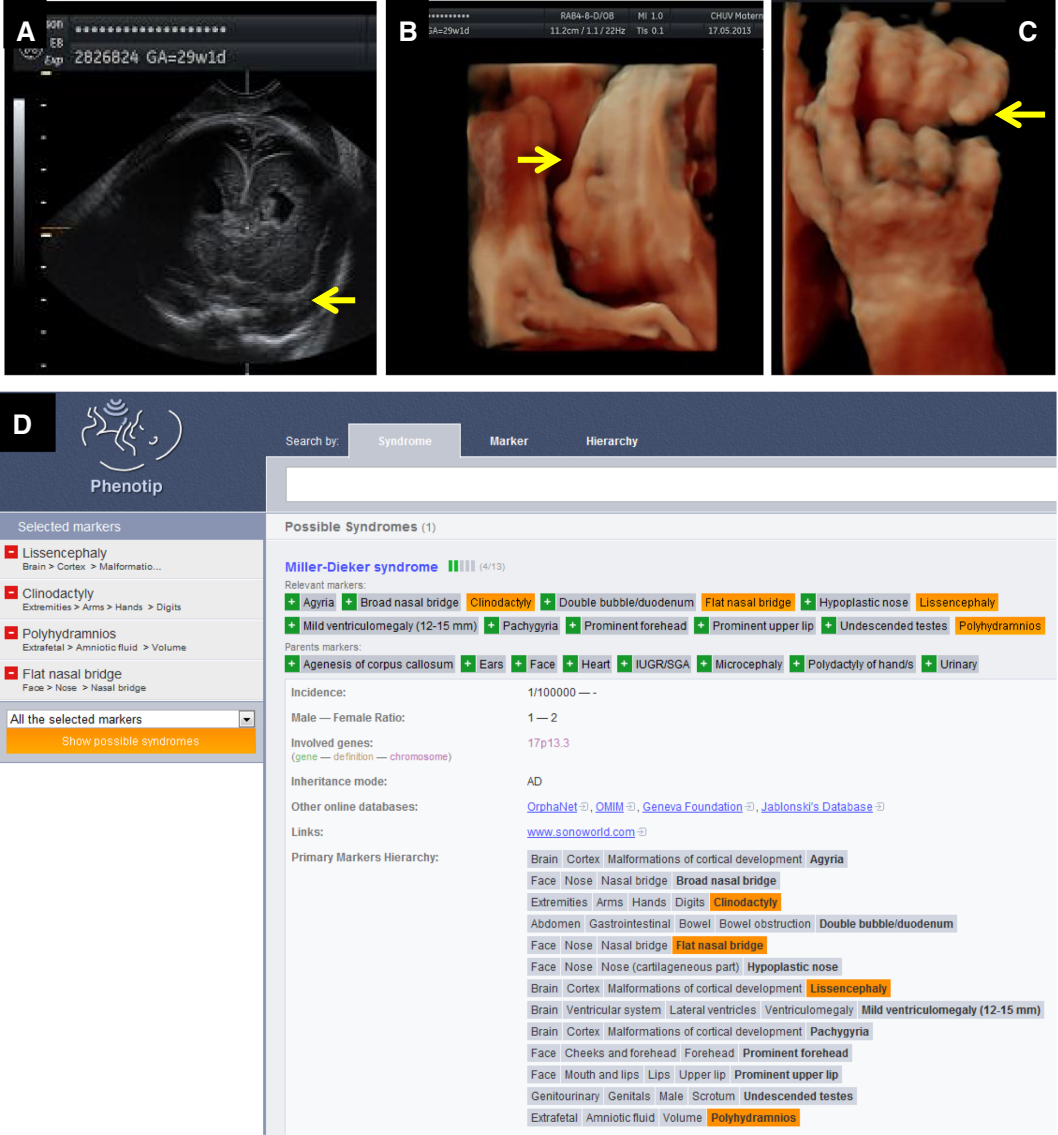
A 28 years old primigravida patient was admitted at 29 weeks gestation for short cervix and abdominal pain. Serologies, 1^st^ trimester screening and anatomy ultrasound at 20 weeks were all normal. On admission, ultrasound showed *polyhydramnios*, *lissencephaly* (A, coronal view), *flat nasal bridge* (B, sagittal 3D-view of the face) and *clinodactyly* (C, 3D-view of the hands). All images kindly provided by Yvan Vial, Lausanne-CHUV, Switzerland. Using the Phenotip.com database, these markers were suspected for a Miller-Dieker syndrome (D). In the Phenotip website, markers can be searched through a hierarchy tree (top right) or the marker search box (top middle). Each selected marker will appear on the left hand side of the screen under “selected marker” after clicking the green button. They can also be removed by clicking the red button. Differential diagnosis will appear after clicking the “show possible syndromes” button on the left hand side of the screen. Amniodrainage was performed, and CGH array confirmed a micro-deletion of locus p13.3 on chromosome 17 including LIS1gene.
